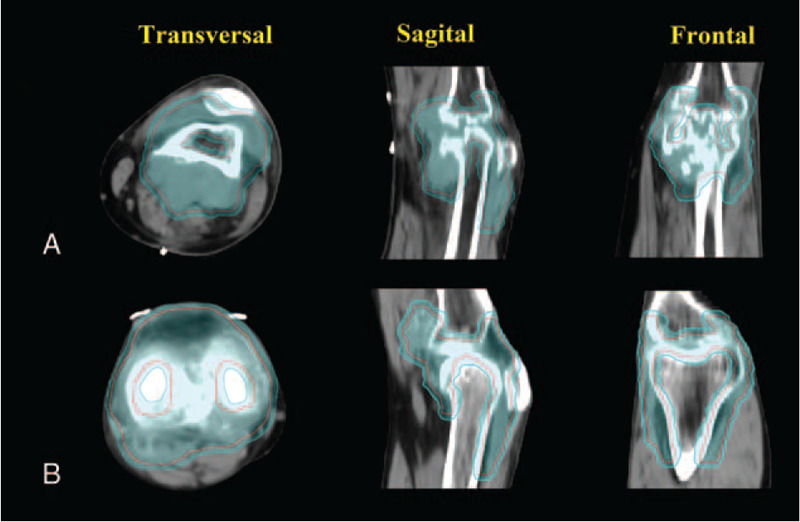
The diagnostic MRI merged with the treatment planning CT. The residual synovial lesions are outlined on the CT and MRI as the gross tumor volume (GTV). The clinical target volume (CTV) includes the whole knee joint cavity and GTV. The planning target volume (PTV) included the CTV with a 0.3 cm isotropic margin.
